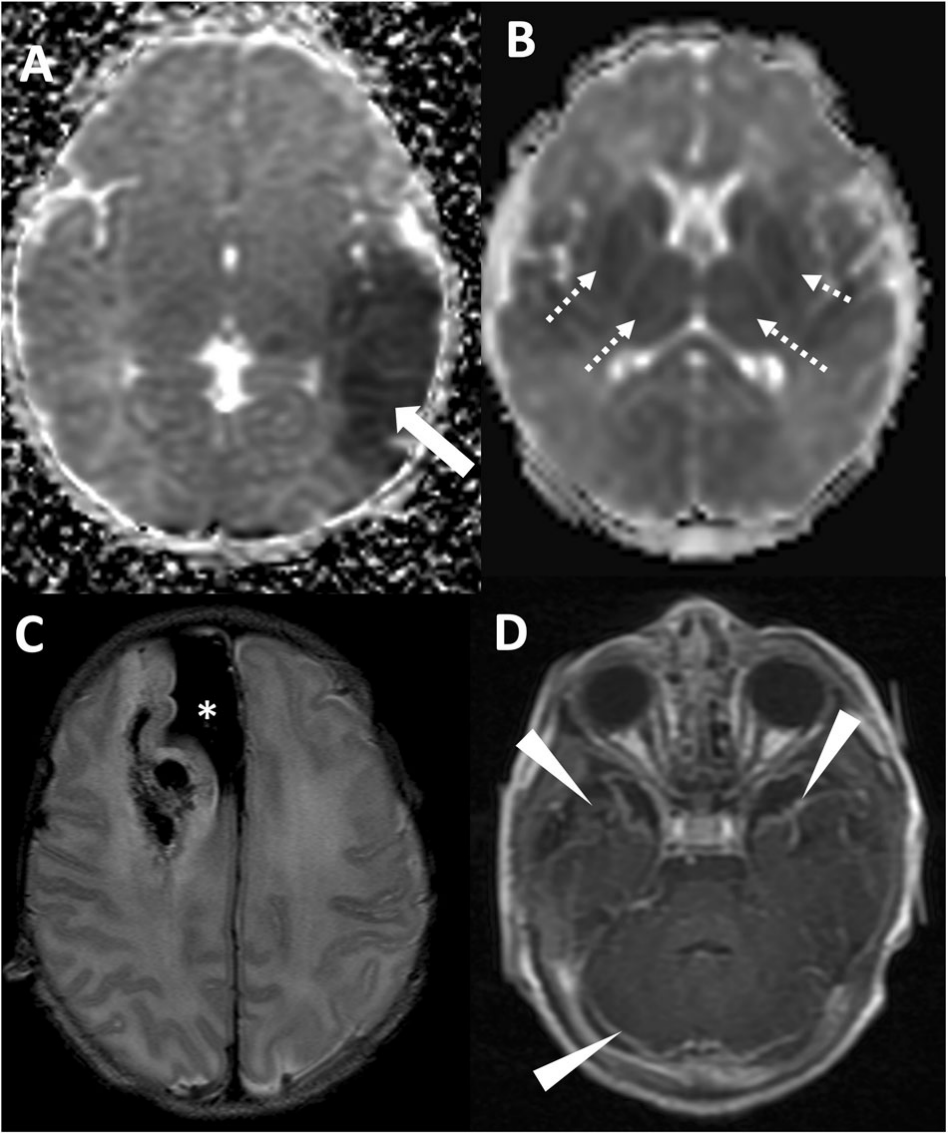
Radiologic diagnoses in the cohort of patients with acute symptomatic seizures. A Axial ADC map demonstrating arterial ischemic stroke in the left middle cerebral artery territory (white arrow). B Axial ADC map demonstrating reduced diffusion in the deep gray nuclei and subcortical white matter, compatible with hypoxic ischemic injury. C Axial T2 weighted imaging demonstrating large subpial hemorrhage in the medial right frontal lobe (asterisk) with associated hemorrhagic injury of the right frontal lobe. D Axial T1-weighted post-contrast imaging demonstrating leptomeningeal enhancement (arrowheads) in the setting of neonatal meningitis/intracranial infection.
